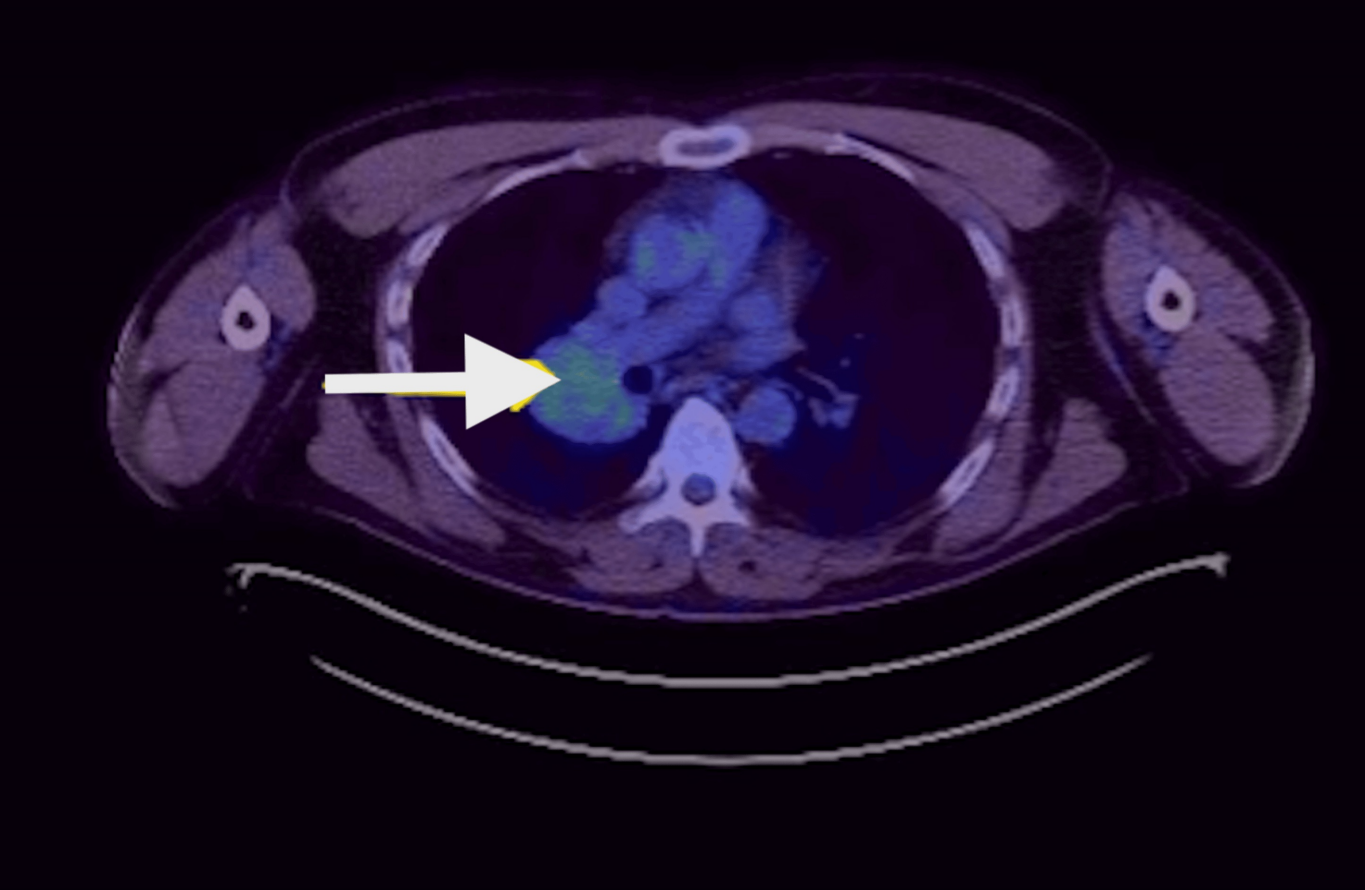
FDG PET-CT in axial view demonstrating low-grade uptake compared to background activity (white arrow). FDG PET: fluorodeoxyglucose positron emission tomography.
